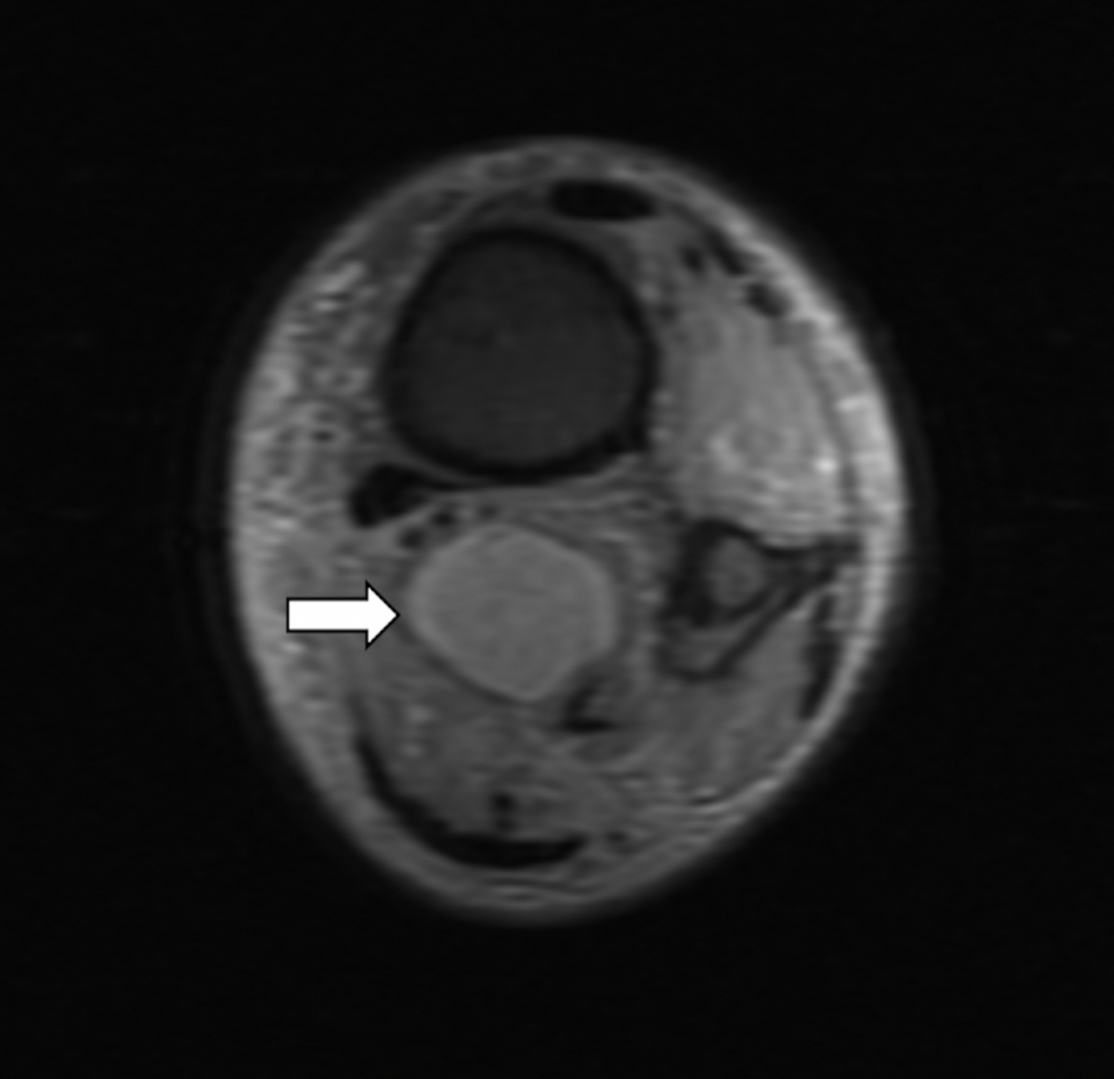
T2 weighted cross-sectional MRI showing the mass arising from posterior tibial nerve
